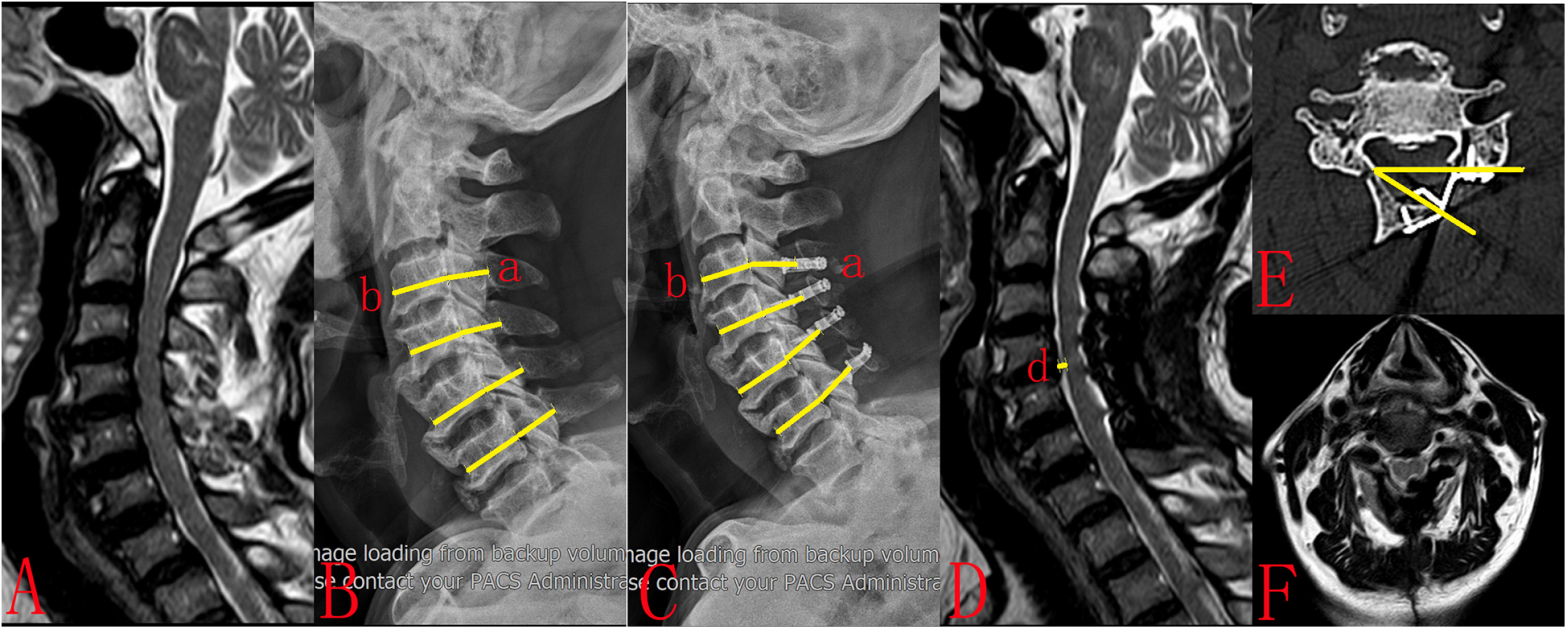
A 67-year-old female patient had been experiencing upper-limb numbness and asthenia for 10 months. (A) Preoperative MRI showed multisegmental spinal cord compression. (B) Preoperative plain radiograph showed cervical canal stenosis (Pavlov ratio = 0.74). (C) The patient underwent cervical unilateral open-door laminoplasty. Centerpiece plates were utilized at the C3-C6 segments (Pavlov ratio = 0.86). (D) Postoperative MRI demonstrated substantial posterior spinal cord drift (*d* = 2.73 mm). (E) Postoperative CT showed that the lamina open angle at C5 was 32.31. However, the ventral prong did not completely adhere to the lamina and instead expanded into the spinal canal. (F) Postoperative MRI demonstrated that the ventral prong in the Centerpiece plate obstruct the spinal cord's backward movement. The ventral prong of Centerpiece plate caused compression on the dorsal side of the spinal cord (D,F).
